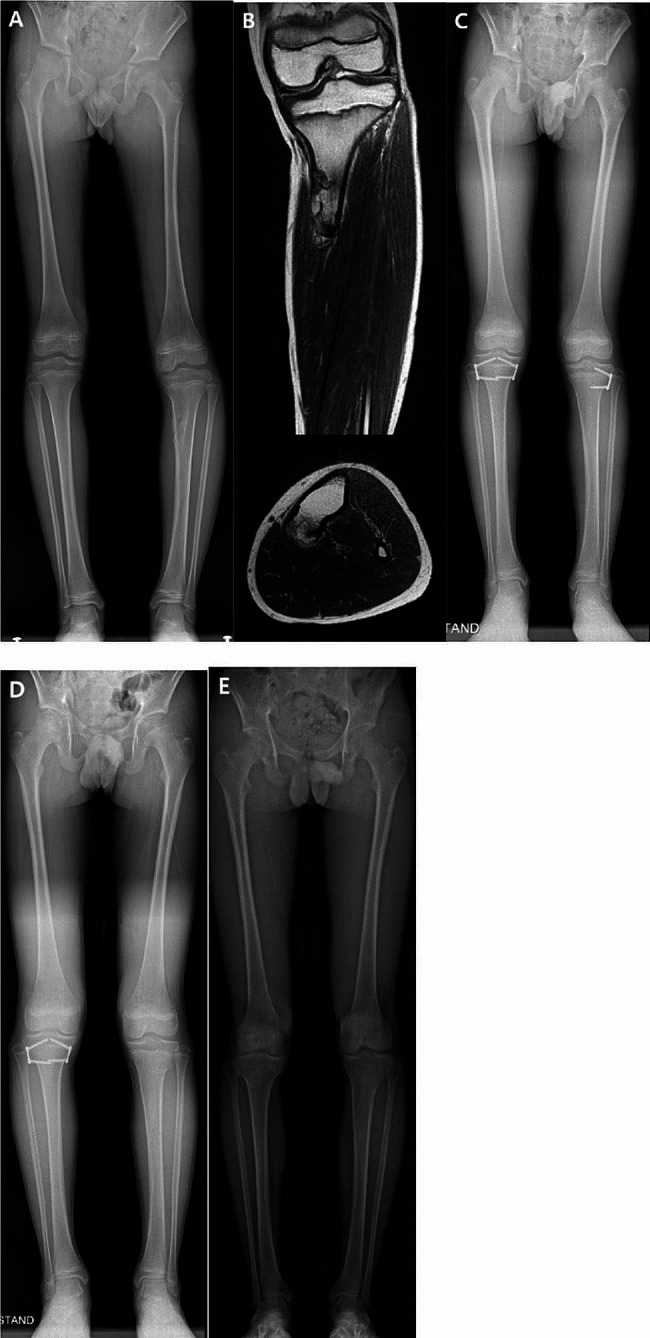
(A) An eleven-year-old boy with a lower limb deformity and genu varum of the left knee. (B) Magnetic resonance imaging showed a sessile-type osteochondroma on the posteromedial aspect of the left tibia. (C) Hemiepiphysiodesis for the left proximal tibia and epiphysiodesis for the right proximal tibia using a tension band plate was performed. (D) The tension band plate was removed after 1 year for the left proximal tibia and after 1 year and 10 months for the right proximal tibia. (E) The final teleroentgenogram showed the correction of LLD and coronal angular deformity
